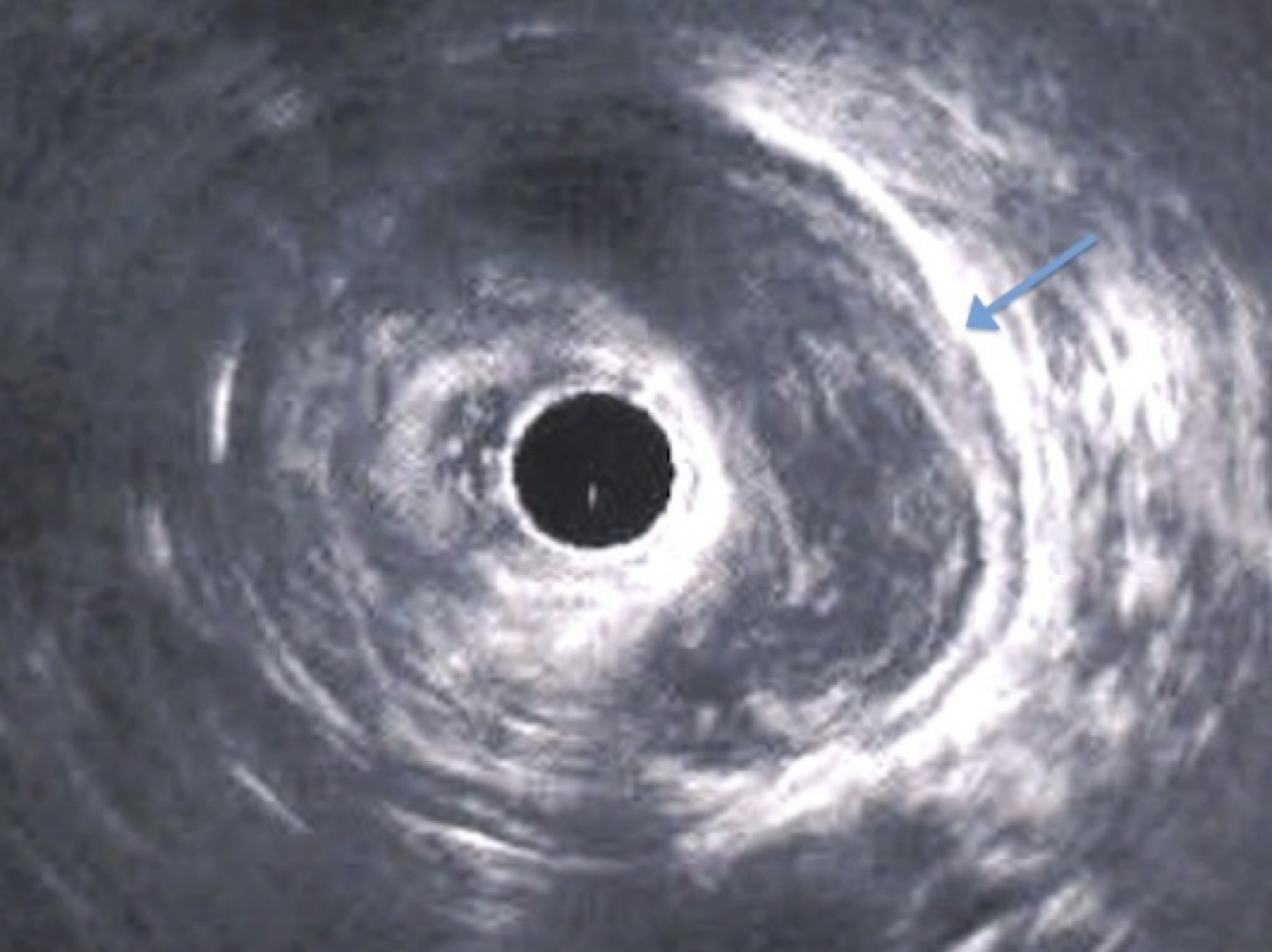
Transrectal ultrasonography Left nonspecific perirectal mass
